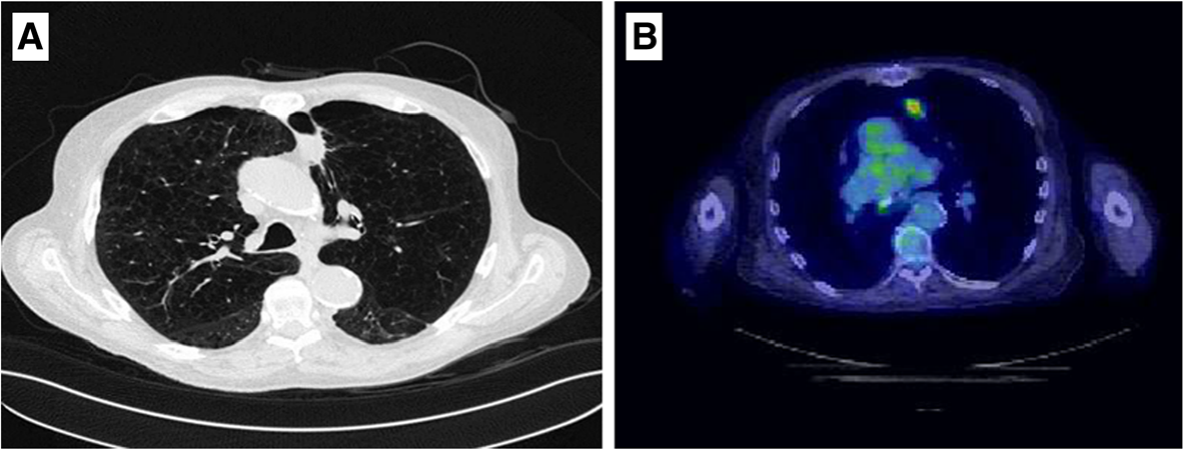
Follow-up CT scan at 30 months post-treatment revealed a 2.5 × 1.9 cm nodule in the previous treatment field with a SUVmax of 5.2.
